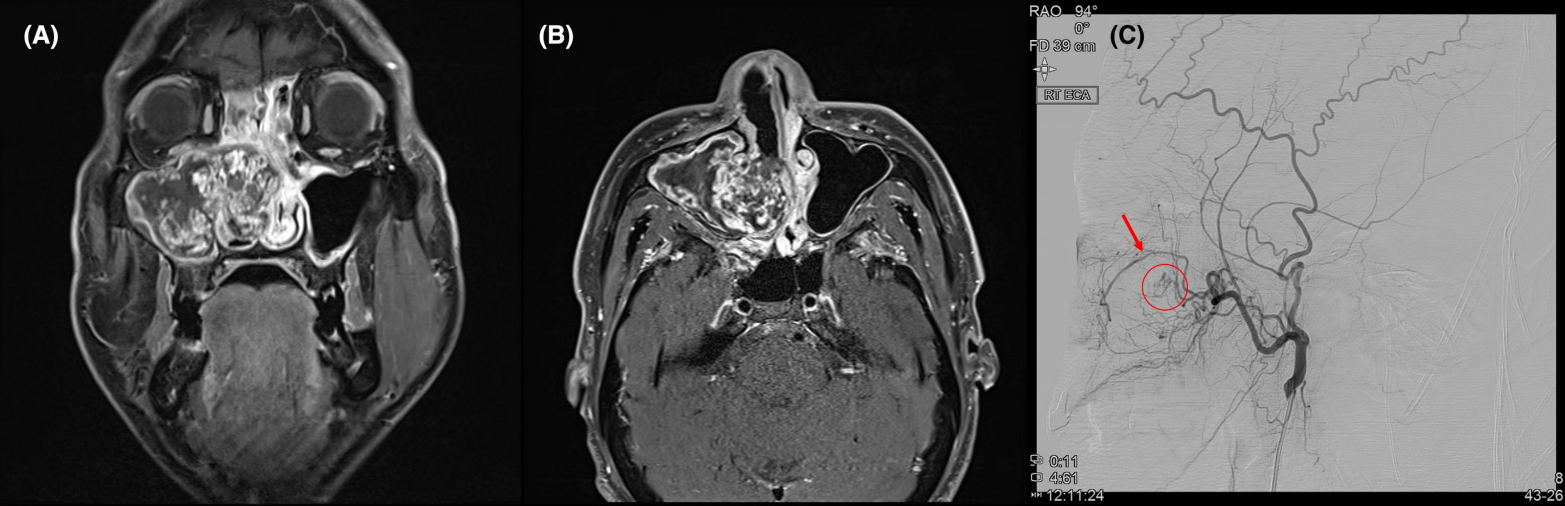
T1‐weighted gadolinium enhanced coronal and axial magnetic resonance images (A) and (B), and angiographic finding (C) of the tumor.
